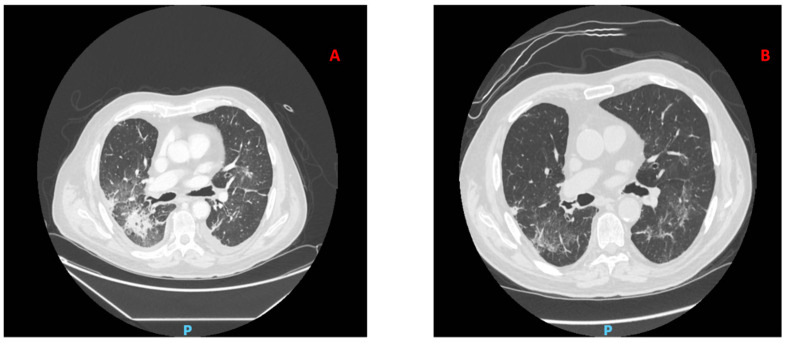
Radiological images (A) CT scan pre-therapy; (B) CT scan 21 days after therapy with L-AmB.
